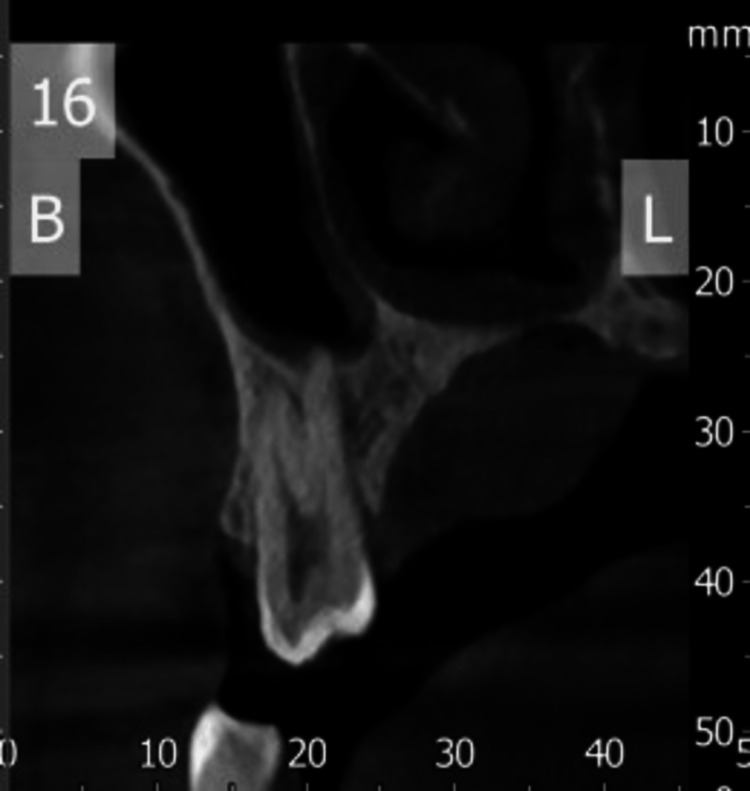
Sectional CBCT section showing scalloping of the palatal cortex
